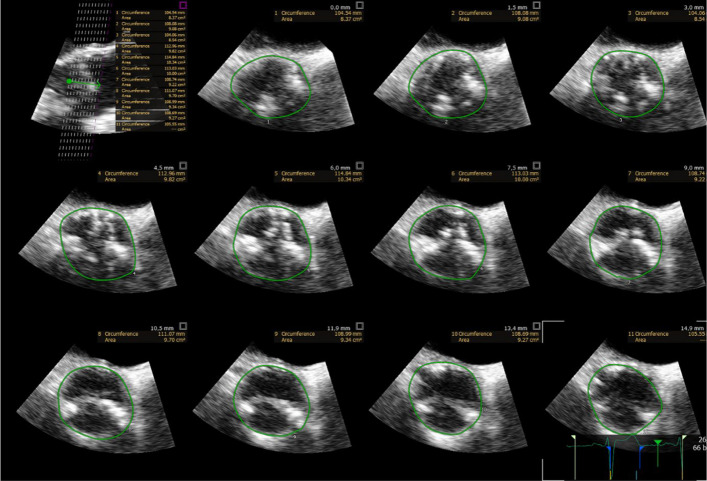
Multiplane rendering of the aortic valve in diastole depicting the intense echocardiography signals corresponding to zones of calcification at different valve levels
